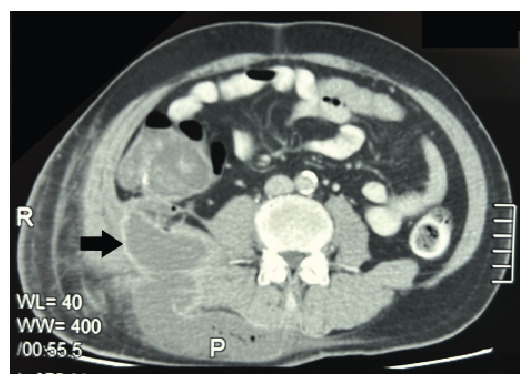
CT scan of the abdomen and pelvis showing a collection in the right retroperitoneal region involving the right quadratus lumborum communicating with a cecal tumor.
